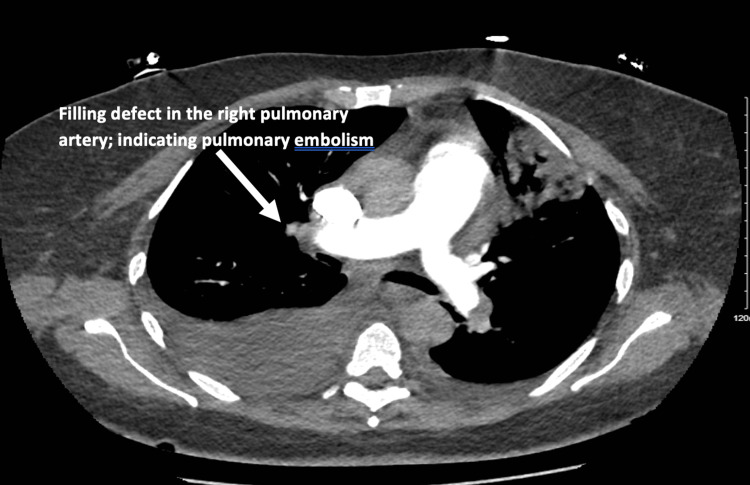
CT pulmonary angiography showing multifocal pulmonary emboli involving the segmental and subsegmental pulmonary arterial vasculature and bilateral pleural effusion (arrow). CT: computed tomography
